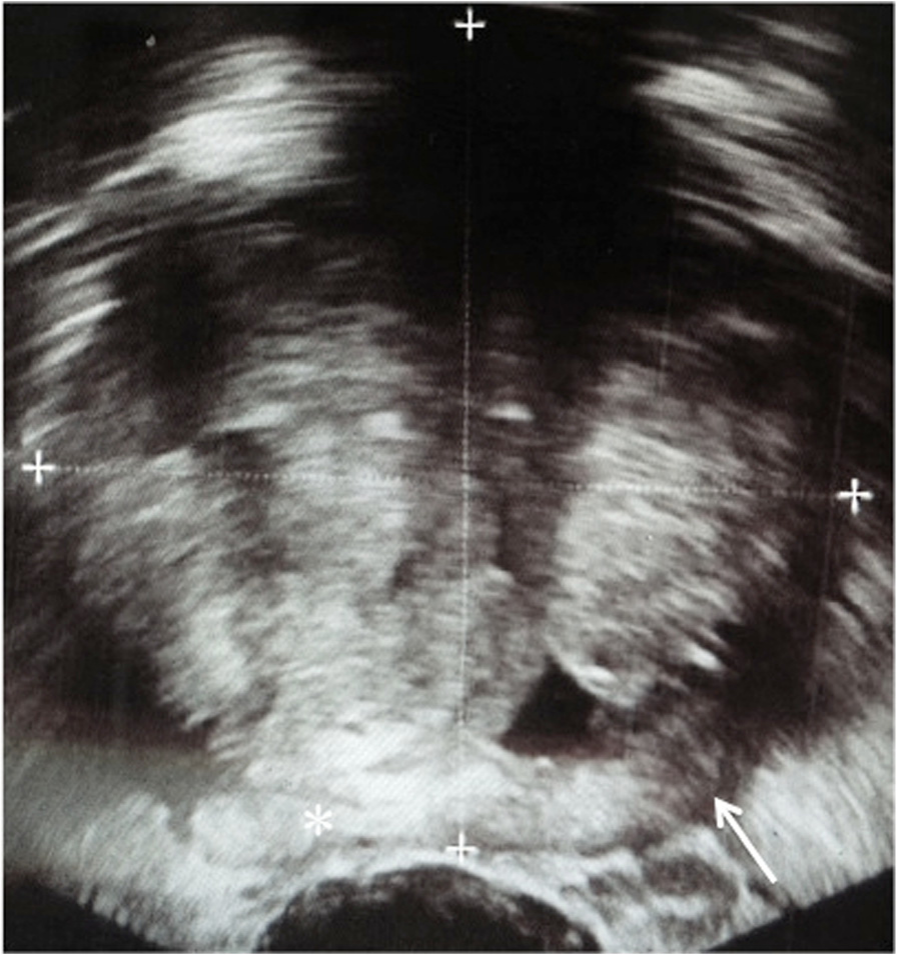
Gray-scale ultrasound image from MR imaging-TRUS fusion biopsy demonstrates the abnormal, hypoechoic lesion in the left seminal vesicle (*arrow*); normal right seminal vesicle for comparison (*asterisk*) and prostate gland (*cross-hairs*)
